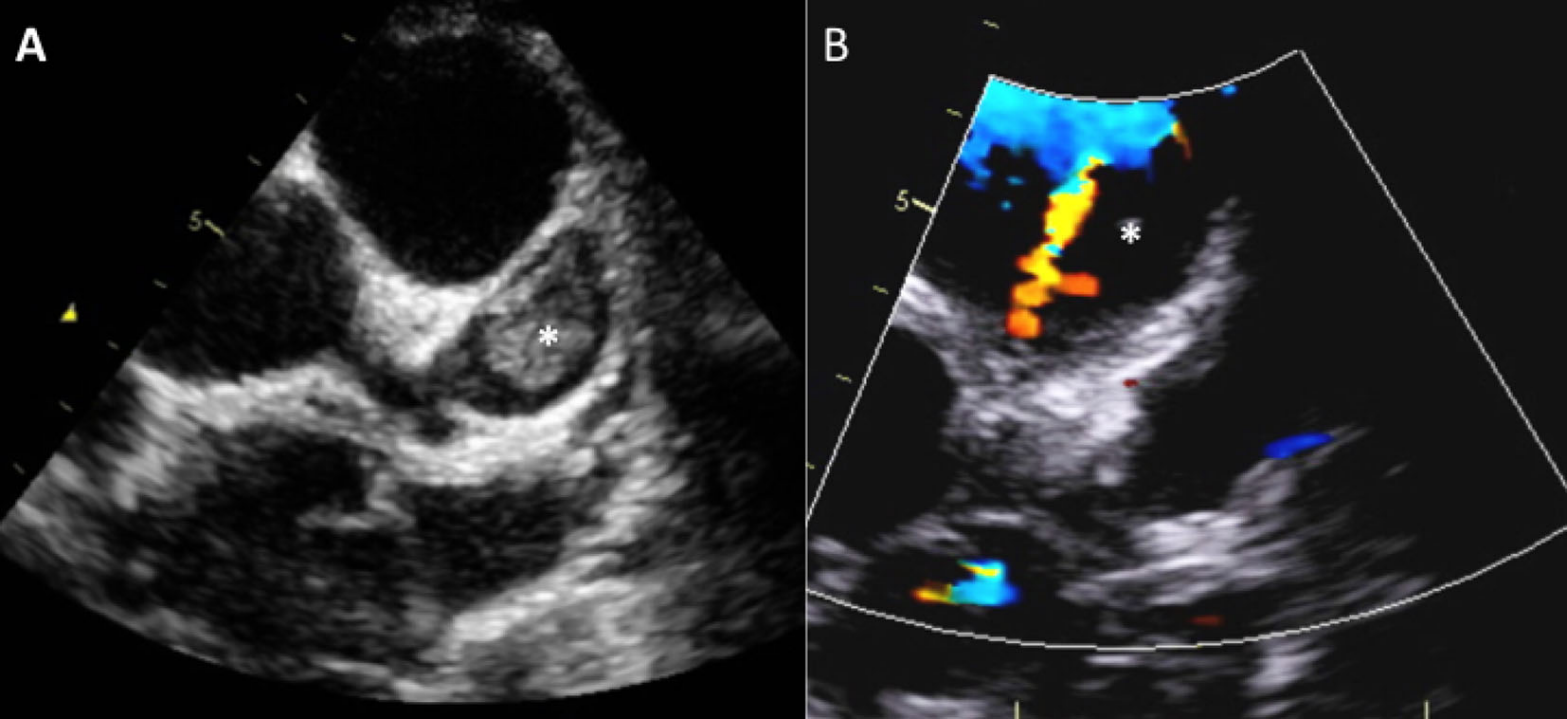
Evidence of acute thrombus in LAD seen on echocardiography. A) Thrombus (asterisk) within the proximal portion of the large LAD aneurysm. B) Accelerating narrowed diastolic flow jet around the peripheral rim of the thrombus. LAD, left anterior descending.
